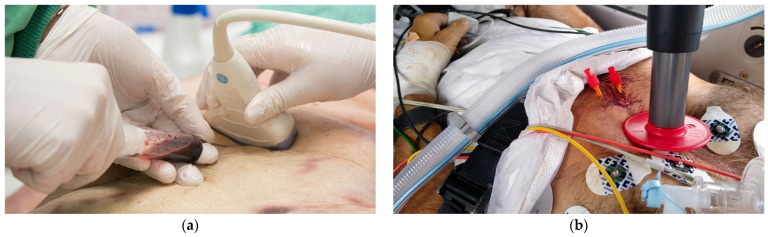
(a) Ultrasound-guided pericardiocentesis in a cadaveric model: The convex-array transducer is placed subxiphoidal to obtain a subcostal window 4-chamber view (S4CH) in order to guide the needle precisely under sight and thus achieve higher success rates; (b) Helicopter transport of a patient in sustained return of spontaneous circulation (ROSC) after ultrasound-guided relief of pericardial effusion in the field by the catheter-over-needle technique. Catheters have been left in place. An automated mechanical cardiopulmonary resuscitation (mCPR) device has been applied precautionarily.
